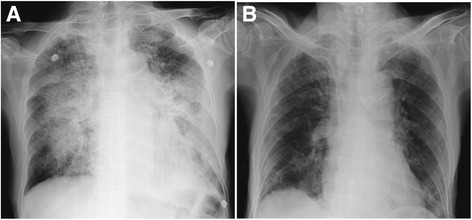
Follow-up chest X-rays. Chest X-ray at hospital day 2 showing rapid progression of ground glass opacities to consolidation (a). After administration of ribavirin, lesions were resolved at hospital day 27 (b)
